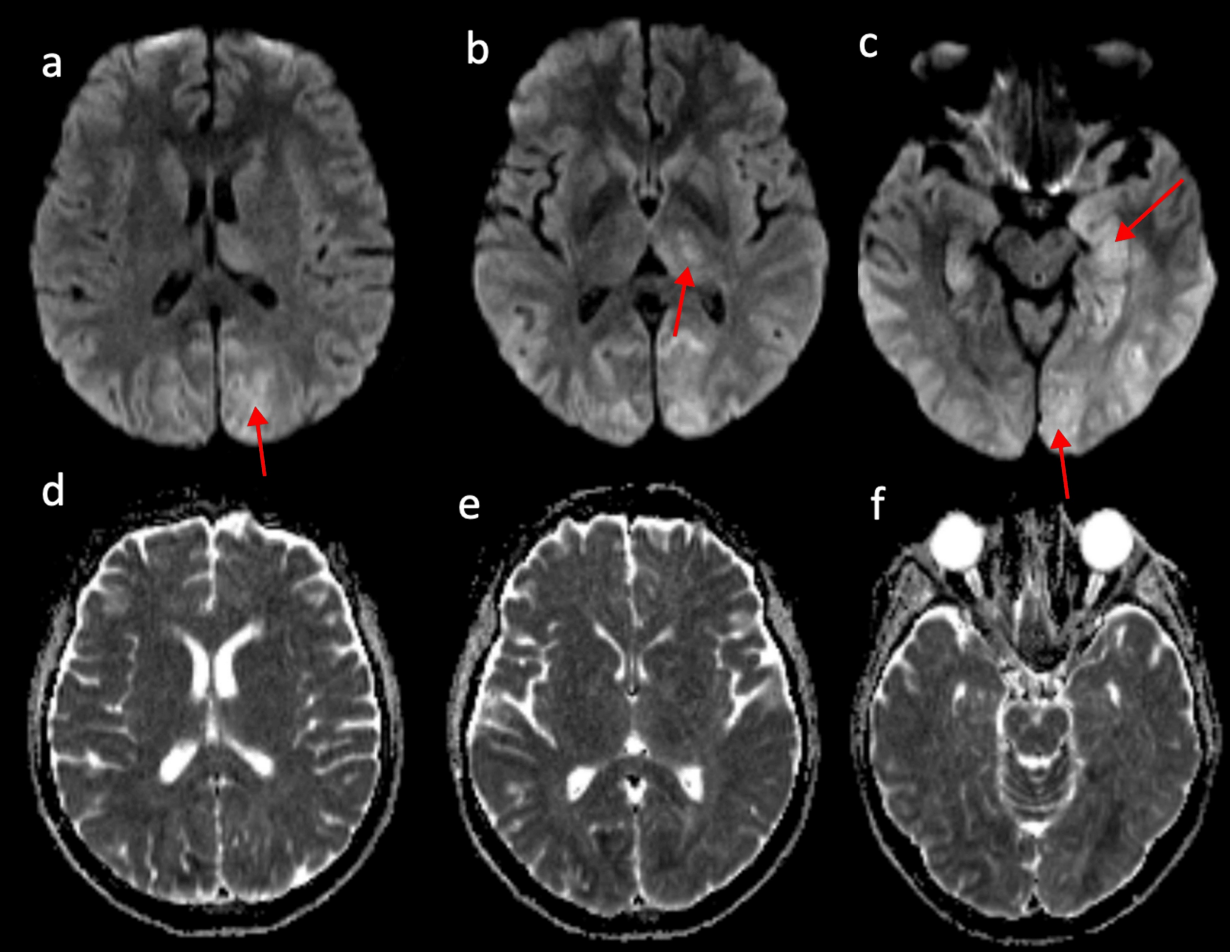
Axial DWI MR images (a,b,c) and ADC map (d,e,f) at the onset. These images reveal acute ischemic lesions in the posterior regions of both cerebral hemispheres, indicative of Posterior Reversible Encephalopathy Syndrome. The DWI images display high signal in the cortex and subcortical white matter of the occipital, parietal, and temporal lobes. Additionally, high signals are noted in the left thalamus and the right part of the splenium of the corpus callosum. The low signal areas on the ADC images reflect cytotoxic edema within vasogenic edema. DWI: Diffusion-Weighted Imaging; ADC: Apparent Diffusion Coefficient
